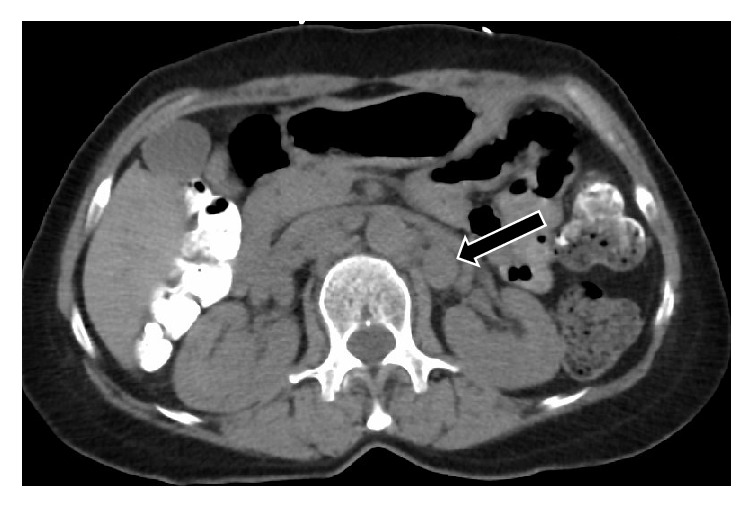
CT of the chest, abdomen, and pelvis performed without contrast (due to allergy) to evaluate for primary malignancy. The only finding was retroperitoneal lymphadenopathy notably of the left para-aortic nodes posterior to the renal vein (arrow).
